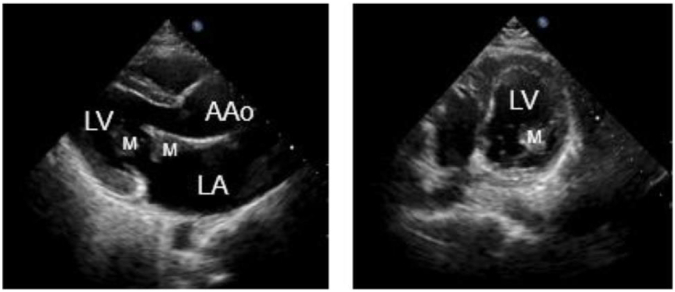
Ultrasound examination of arterial endometrial sarcoma.
